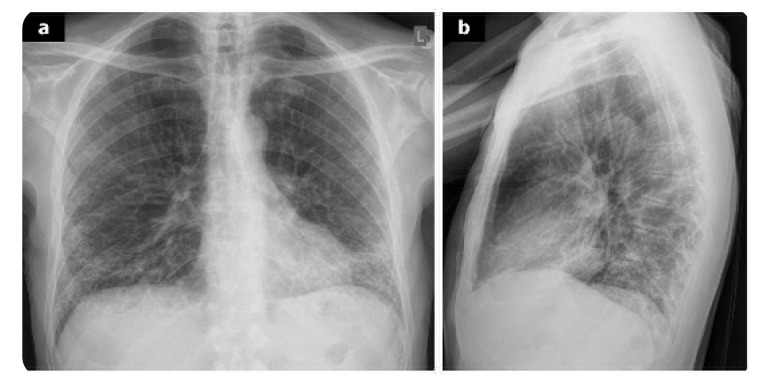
A 65-year old smoker with seropositive RA and dyspnoea on exertion. Antero-posterior (a) and lateral view (b) of x-rays show severe reticular opacities affecting the middle and lower zones of the lung.
